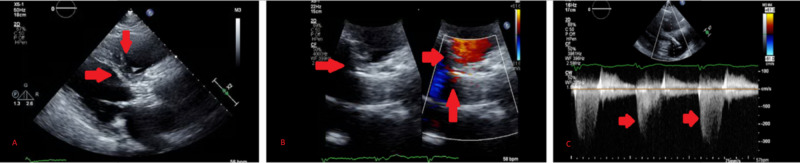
Echocardiogram post transcatheter aortic valve replacement (A, B) Parasternal long-axis view with red arrows indicating normally opening bioprosthetic aortic valve in systole which is also demonstrated by normal color flow Doppler. (C) Normal gradients across the replaced bioprosthetic aortic valve indicated by red arrows corresponding to a normally functioning valve.
